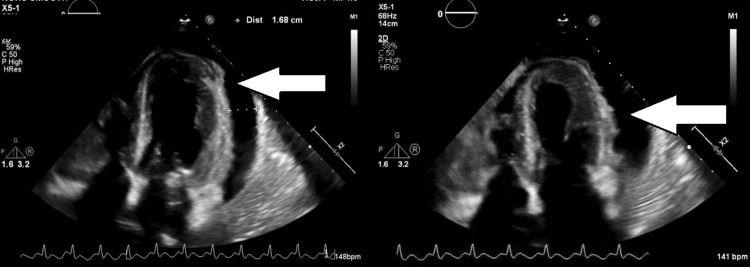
Four-chamber view of the echocardiogram showing a moderate-sized pericardial effusion, and a left ventricular apical anterior myocardium with Echo density suspicious for cardiac mass. Arrows are pointing towards the pericardial effusion
